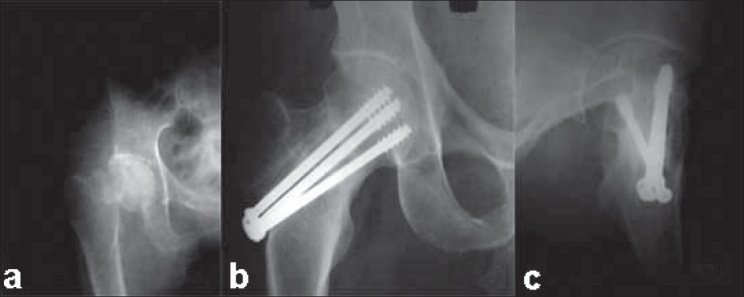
(a) Anteroposterior view of the right hip of a three months old femoral neck fractre, fixed with cancellous screw and nonvascularized fibular graft. Follow-up (anteroposterior (b) and lateral view (c)) radiograph at 18 months showing fracture union and graft incorporation.
